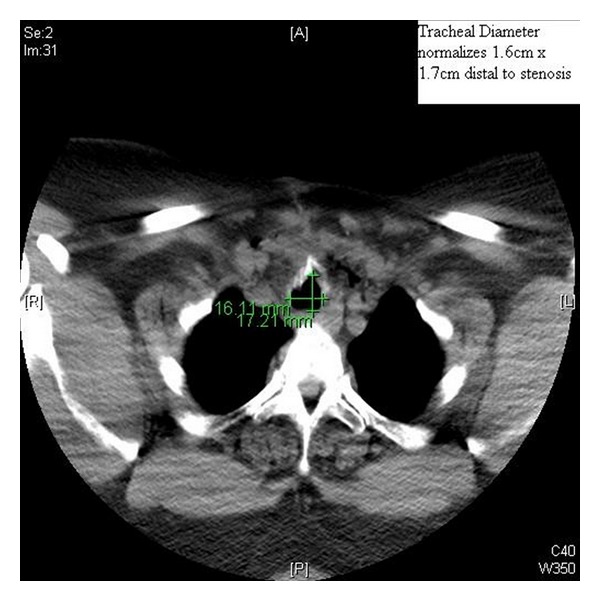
CT SCAN of tracheal diameter.
